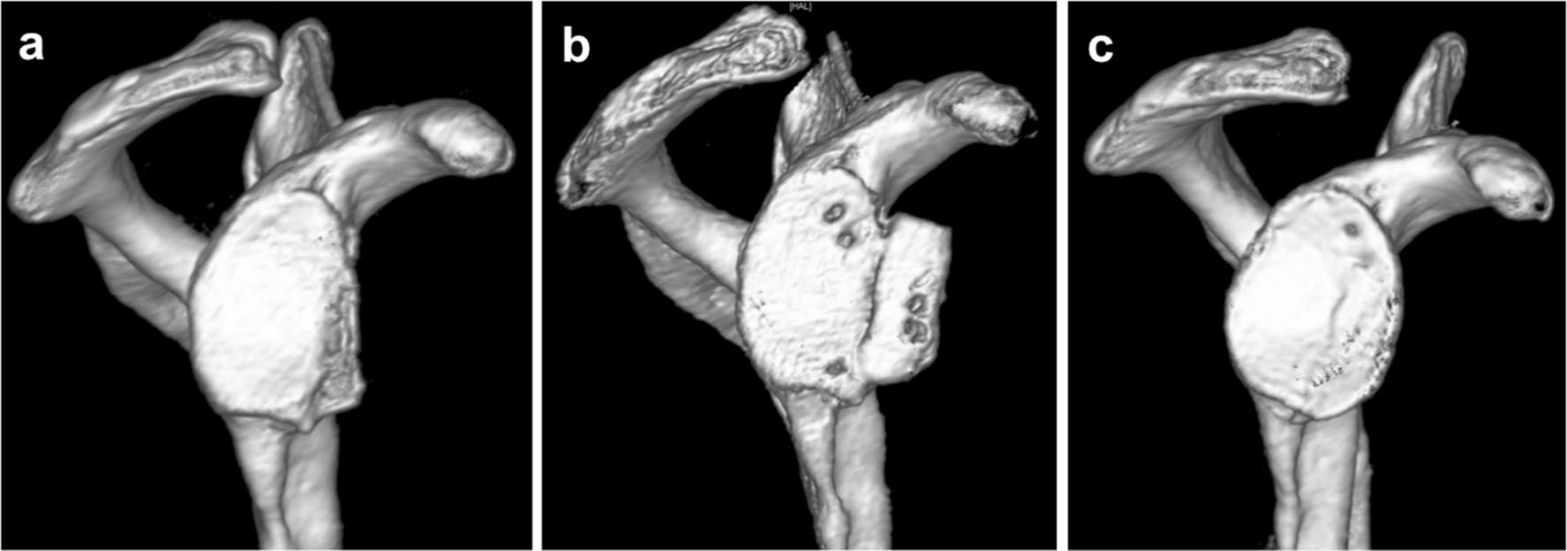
3D-CT-reconstruction of the scapula with subtraction of the humeral head (a) preoperative, (b) directly postoperative, and (c) at the final follow-up 78.7 months postoperatively displaying the remodeling process of the glenoid
